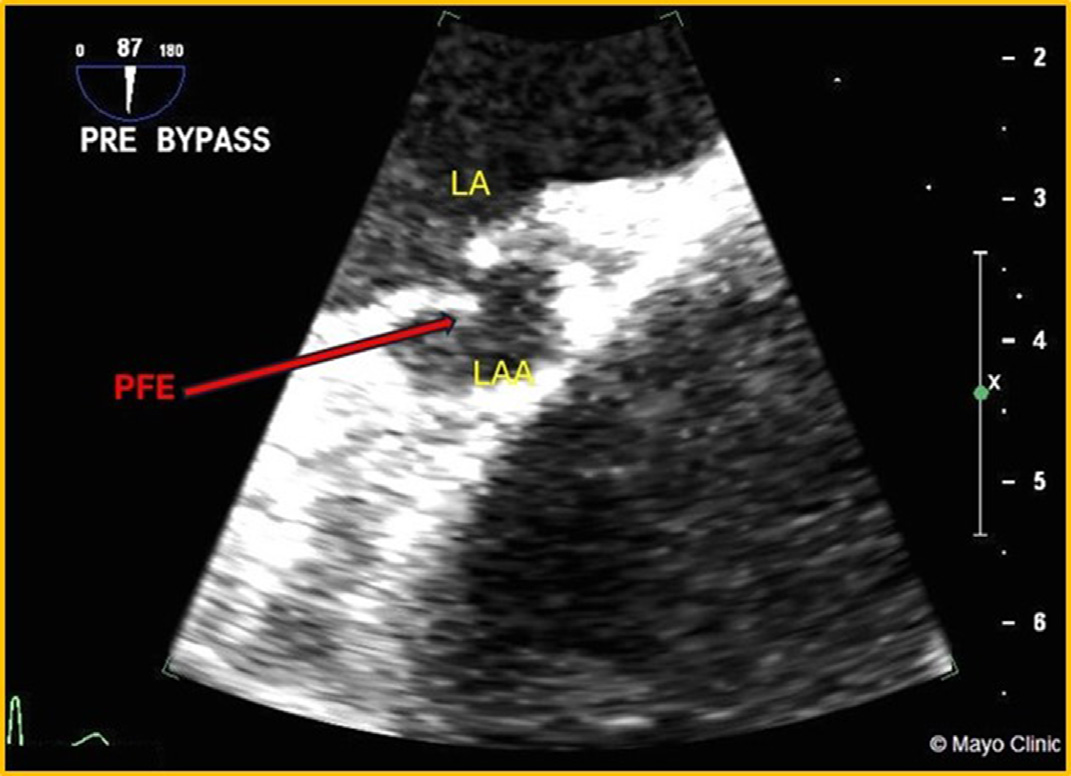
Transesophageal Echo (Left Atrial Appendage Mass-Zoomed) Intraoperative TEE revealed an incidental small mobile fibrinous strand attached to the orifice of the LAA. Abbreviations as in Figure 1.
